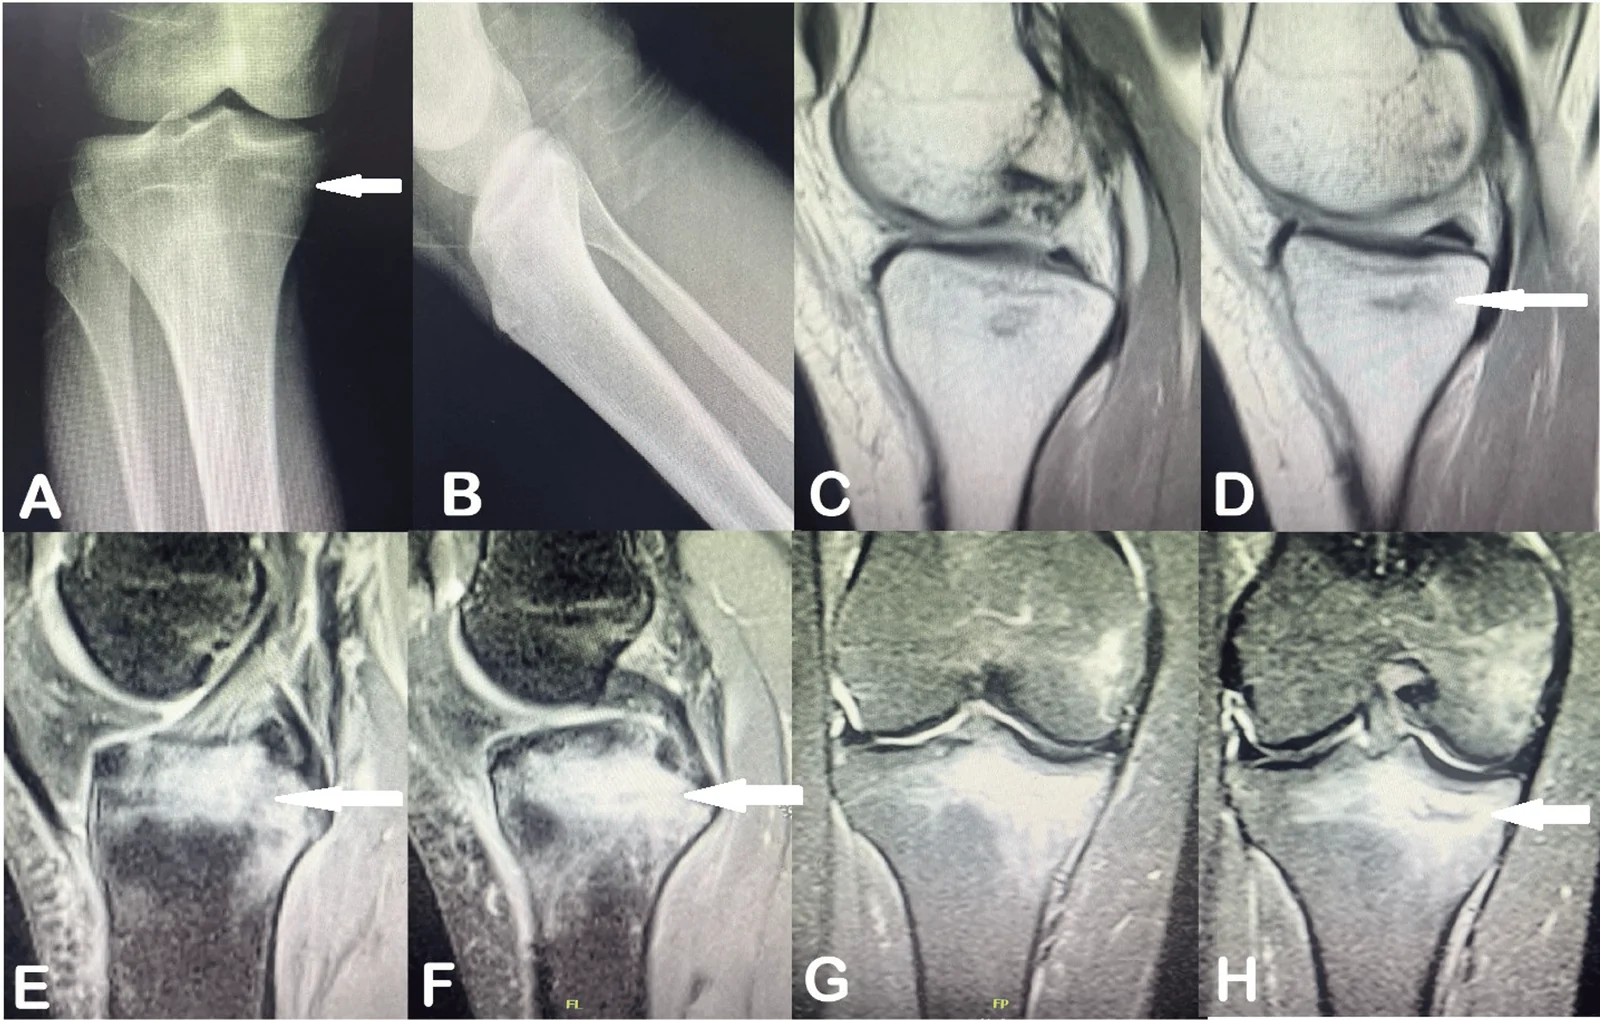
AP and lateral knee radiographs of a 26-year-old female (A, B) AP and lateral knee radiographs obtained at the initial presentation of a 26-year-old female military recruit showing no obvious pathology, with a possible stress fracture line (white arrow). (C, D) T1 sagittal knee MRI images of the same patient showing a fracture line of low signal without obvious signs of bone edema (white arrow). (E-H) T2 sagittal (E+F) and coronal (G+H) knee MRI images showing an obvious fracture line surrounded by significant bone edema representing a stress fracture (white arrow). AP, anteroposterior.
